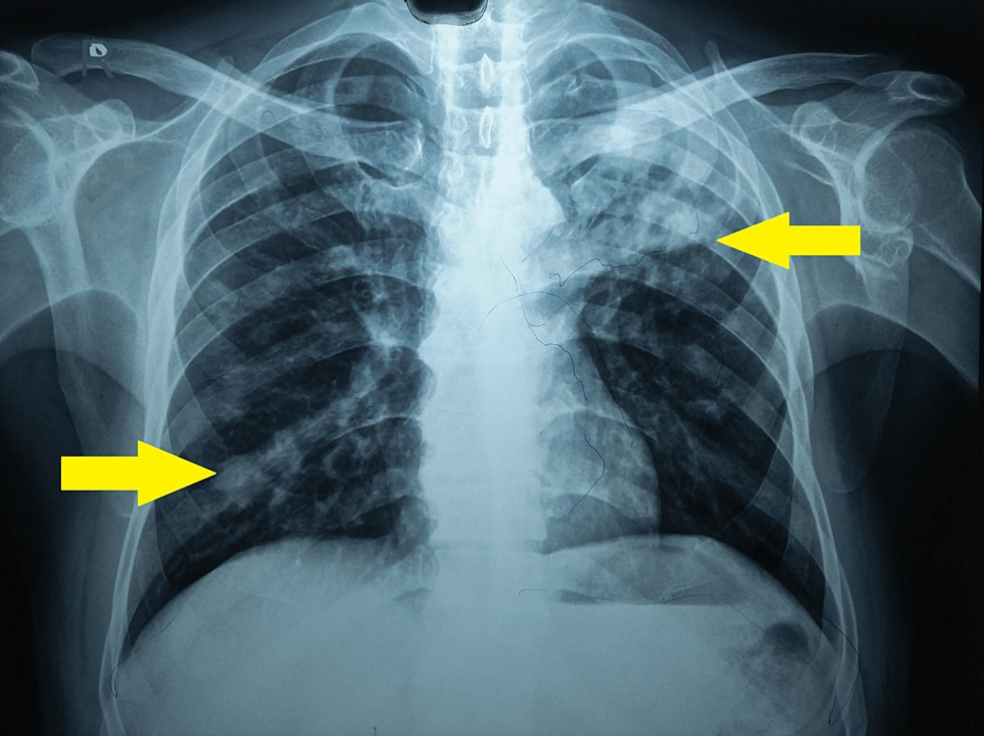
An antero-posterior chest radiograph showing bilateral involvement with a solid oval mass within a left upper lobe cavity and right lower lobe consolidation.
